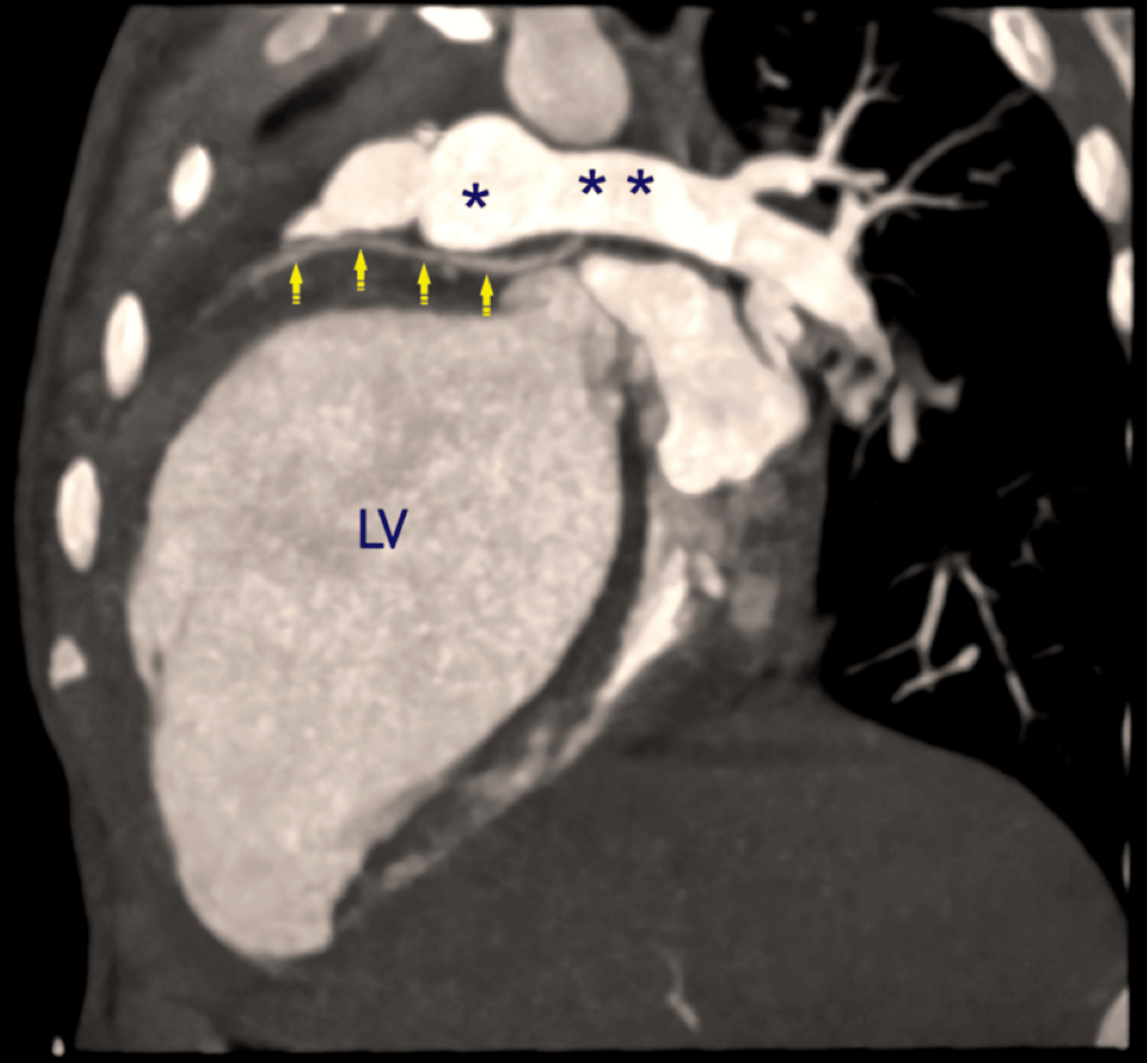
The oblique sagittal maximum intensity projection (MIP) reconstruction of CT angiography reveals that the LCA started abnormally from the undersurface of the RPA (ALCARPA, yellow arrows) with a dilated left ventricle (LV). , RPA, right pulmonary artery; *, MPA, main pulmonary artery; CT, computed tomography Image Credits: Dr. Sachin Talwar
